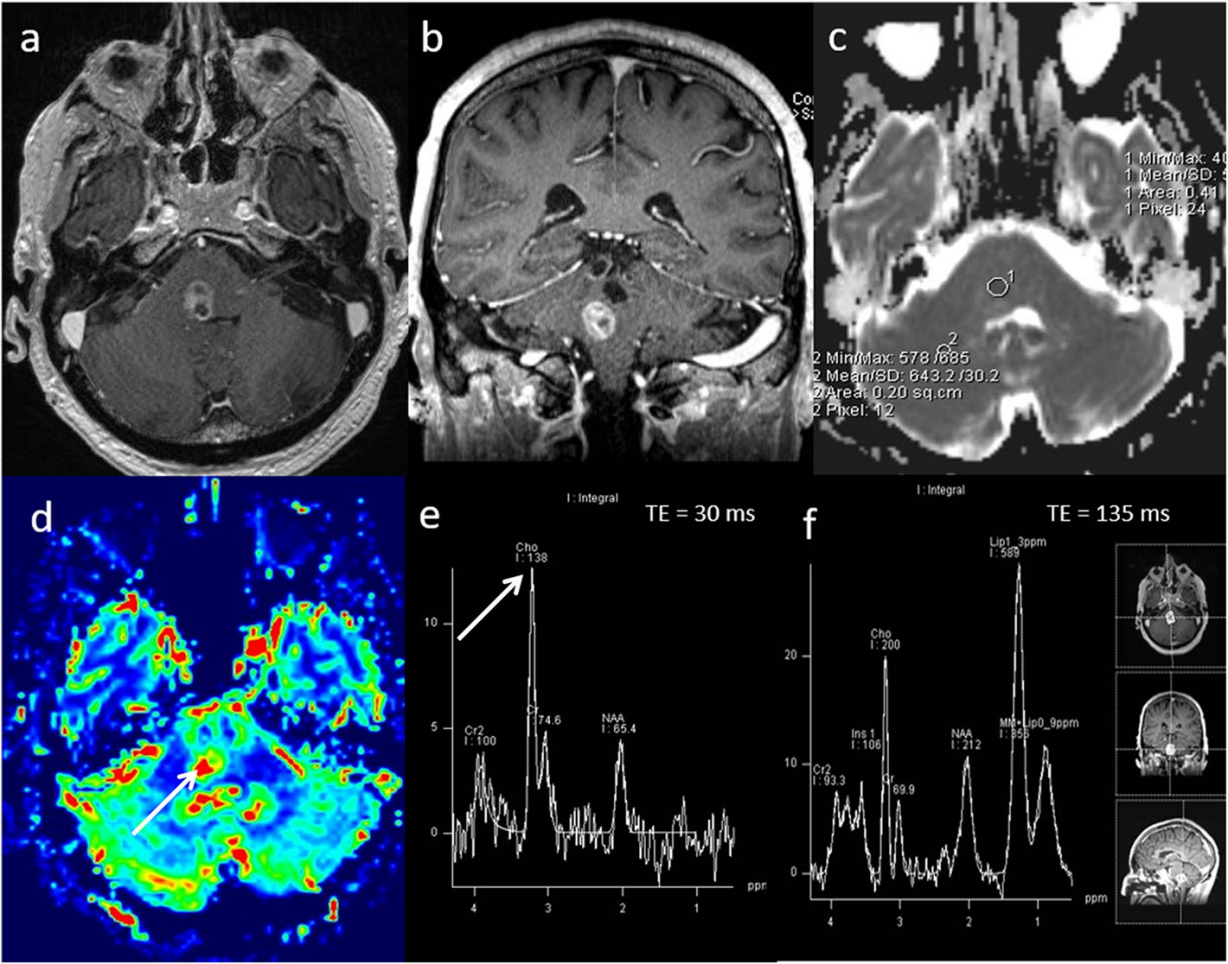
High-grade glioma. Conventional MRI: a, b Axial and coronal post-contrast T1W sequences, showing a well-defined lesion at the ponto-medullary junction. Multiparametric MRI: c ADC map demonstrates low ADC (590 × 10^−6^ mm^2^ s^−1^). d PWI shows high perfusion (rCBV 2.8, arrow). e, f MRS shows a high Cho/Cr ratio (2.9, arrow), low NAA/Cr ratio and presence of lipid peaks. MRI findings of a low ADC (< 1000 × 10^−6^ mm^2^ s^−1^), high rCBV (> 2) and high Cho/Cr ratio (> 1.8) are consistent with a high-grade glioma rather than a granuloma or abscess. The presence of high choline levels in the perilesional area (not shown) favour high-grade glioma over a metastatic lesion. In this patient, an initial biopsy was inconclusive and as a result of the multiparametric MRI findings, a decision to undergo further biopsy was overturned. The patient underwent radiotherapy for presumed glioblastoma
